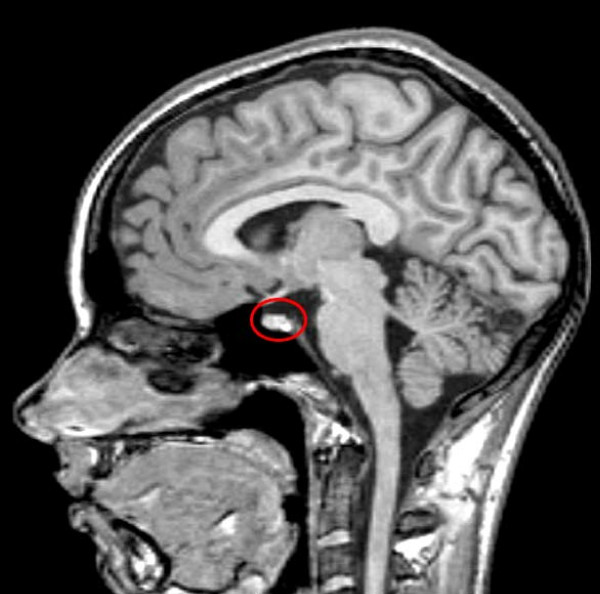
T1-weighted MRI of her pituitary with contrast showing normal appearances of pituitary (circled in red).
